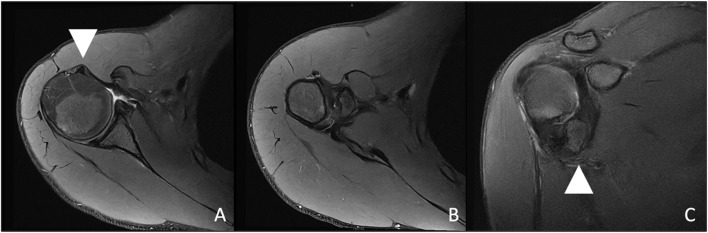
Axillary (A-B) and coronal (C) MRI views of a 19-year-old male with a lesser tuberosity avulsion fracture, subluxated biceps tendon (A), axillary nerve (C) highlighted by the triangular marker
